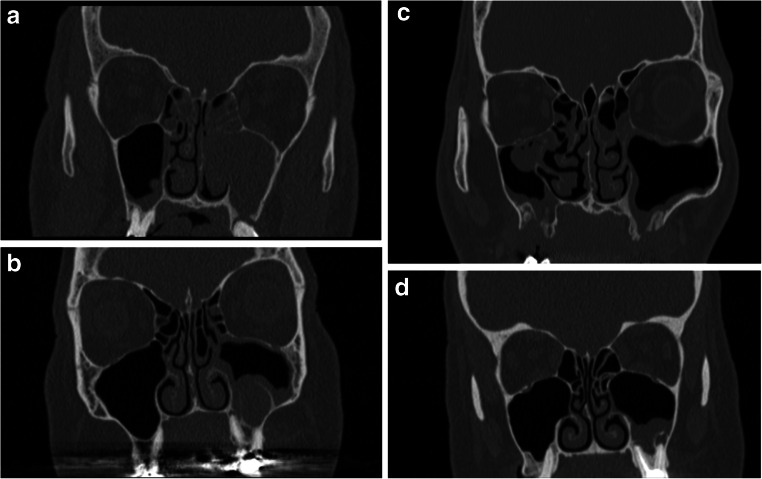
Coronal computed tomography views of four likely chronic odontogenic sinusitis patients. The pathological maxillary teeth findings were examined based on the radiological reports. a Apical periodontitis in the left first maxillary molar. An oro-antral fistula following extraction of the left second maxillary molar and an infected, unerupted left third maxillary molar connected to the previous fistula (not shown). b Deep filling and untreated roots in the left first maxillary molar. A cystic lesion extends from the buccal roots to the sinus and a small apical periodontitis surrounds the palatal root. c Bilateral oro-antral fistulas following extractions of the left first and third maxillary molars and right second and third maxillary molars. Defects in the maxillary sinus floor bone, maximum size 15 mm × 9 mm on the right side and 7 mm × 9 mm on the left side. d Apical periodontitis in all of the roots of a root canal treated left first maxillary molar and bone defects from the buccal roots to the maxillary sinus floor
